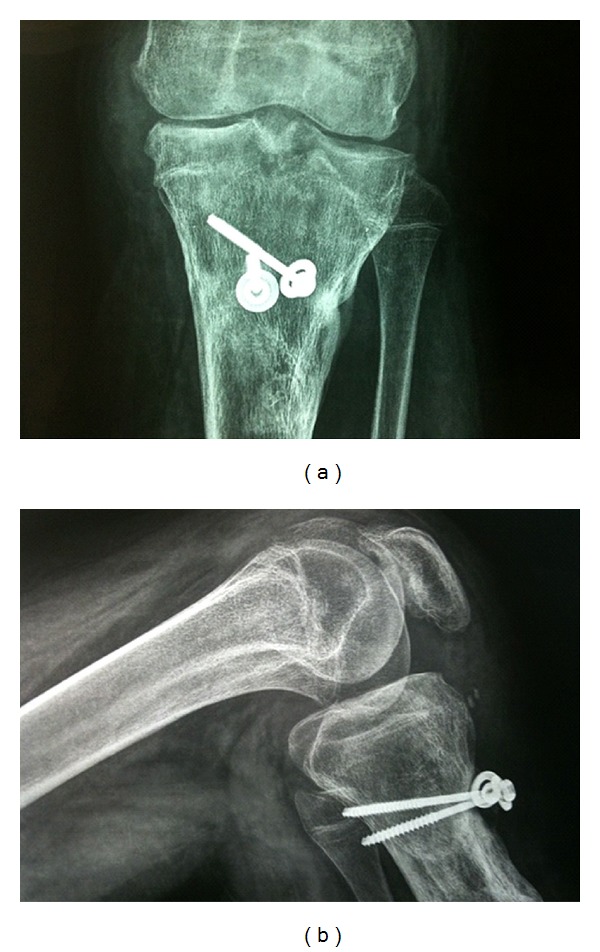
X-rays of the left knee two years after the procedure.
